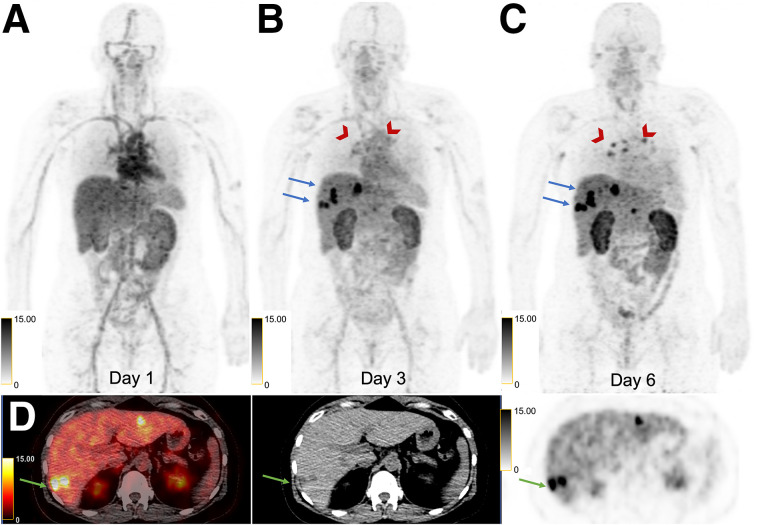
50-y-old woman with HER2-positive mBC and known right lung, thoracic nodal, and liver metastases. (A–C) Sequential maximum-intensity projection PET images at 1 d (A), 3 d (B), and 6 d (C) after administration of ^89^Zr-ss-pertuzumab demonstrating gradually increasing uptake in thoracic nodes (arrowheads) and hepatic lesions (arrows) over time. Decreasing blood pool and slightly decreasing liver background uptake was observed on serial imaging, with increasing kidney uptake. (D) Axial ^89^Zr-ss-pertuzumab PET/CT, CT, and PET images at 6 d demonstrating a few avid hepatic lesions, of which segment 6 lesion (arrows) was selected for biopsy.
